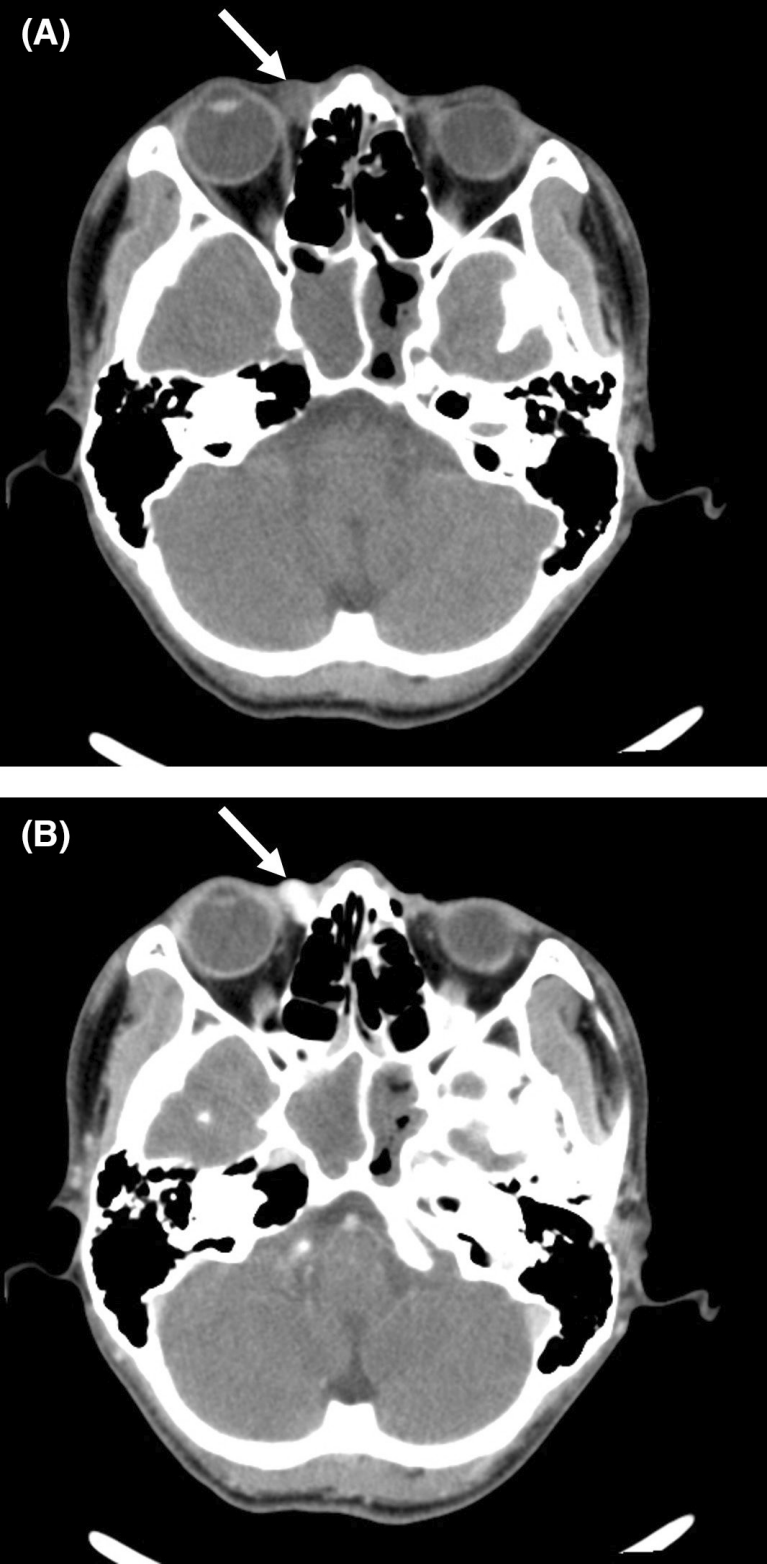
Computed tomography of superficial angiomyxoma. (A) Plain computed tomography showed that the tumor (arrow) under the medial rectus muscle of the right eye was nodular and comparatively defined with a value of 44 HU. (B) Enhanced computed tomography showed that the tumor (arrow) was obviously enhanced with a value of 157 HU–178 HU.
